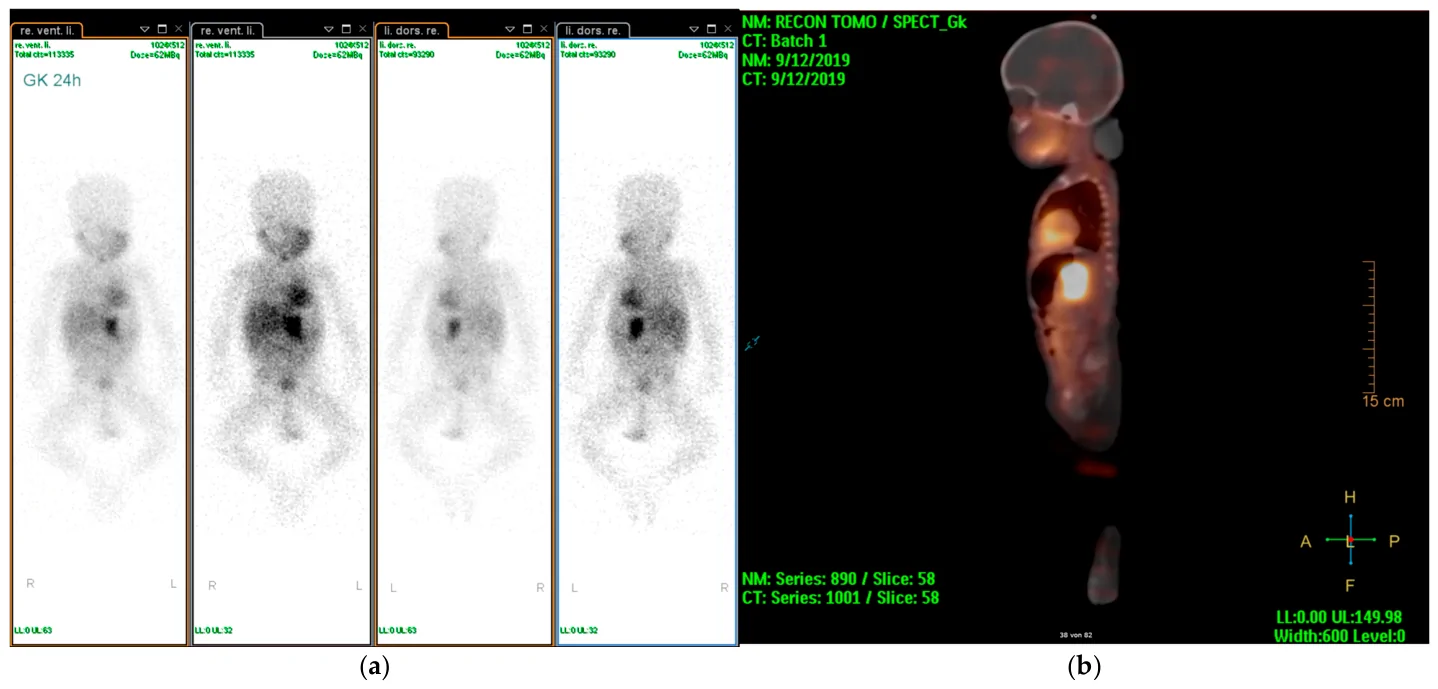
(a,b) Nuclear medicine findings. (a) ^123^I-metaiodobenzylguanidine (MIBG) scintigraphy: tracer uptake in the mandible (anterior and posterior views). (b) SPECT/CT: pathological tracer uptake in the mandible and the left adrenal gland. (Department of Radiation Medicine, Nuclear Medicine, University Hospital Halle; Dr. Ch. Geibig).
